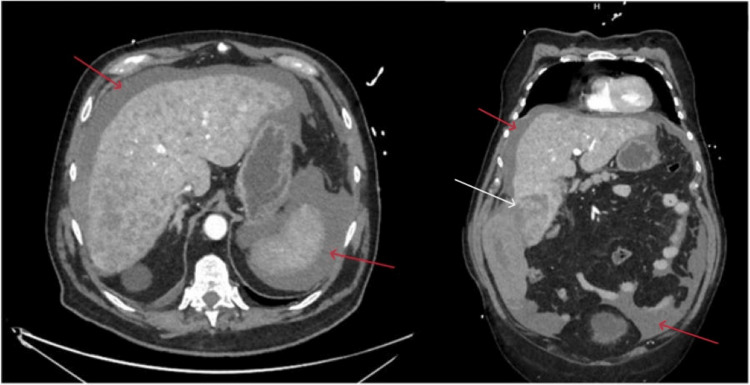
Abdominal CT scan showing hemoperitoneum (red arrows) and evidence of contrast leakage near segment 6 (white arrow)
